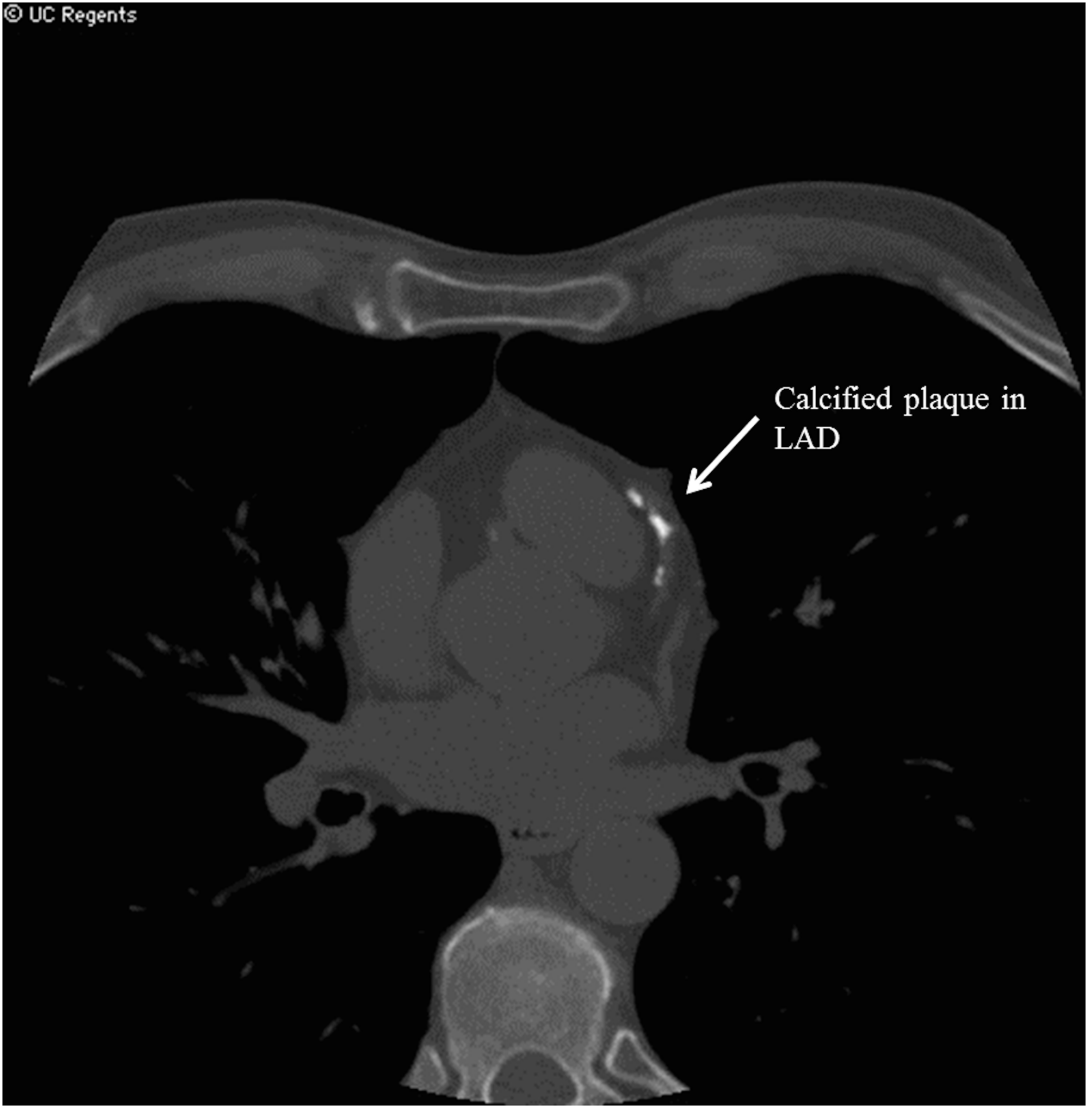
CAC EBCT of a subject with detectable CAC. Abbreviations: Coronary artery calcium (CAC), External beam computed tomography (EBCT).
